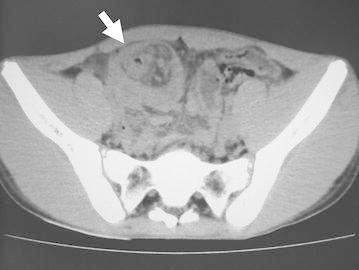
Abdominal CT at previous clinic. This CT was performed 5 weeks before he admitted to our hospital. Retrospectively, intussusception could be pointed out (*arrow*).
